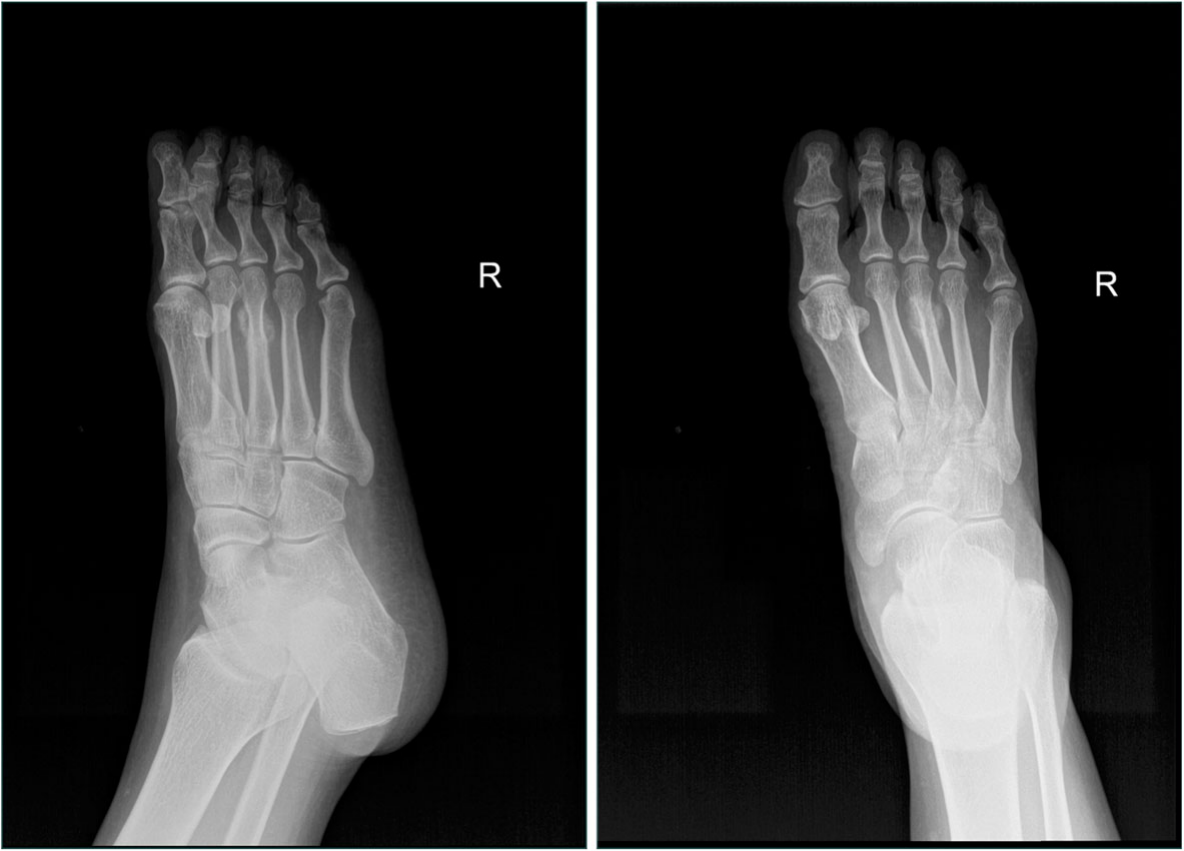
Plain X-rays of the right foot showing the lesion on the neck of the third metatarsal, with narrowing of the medullary cavity, with no visible fracture line
